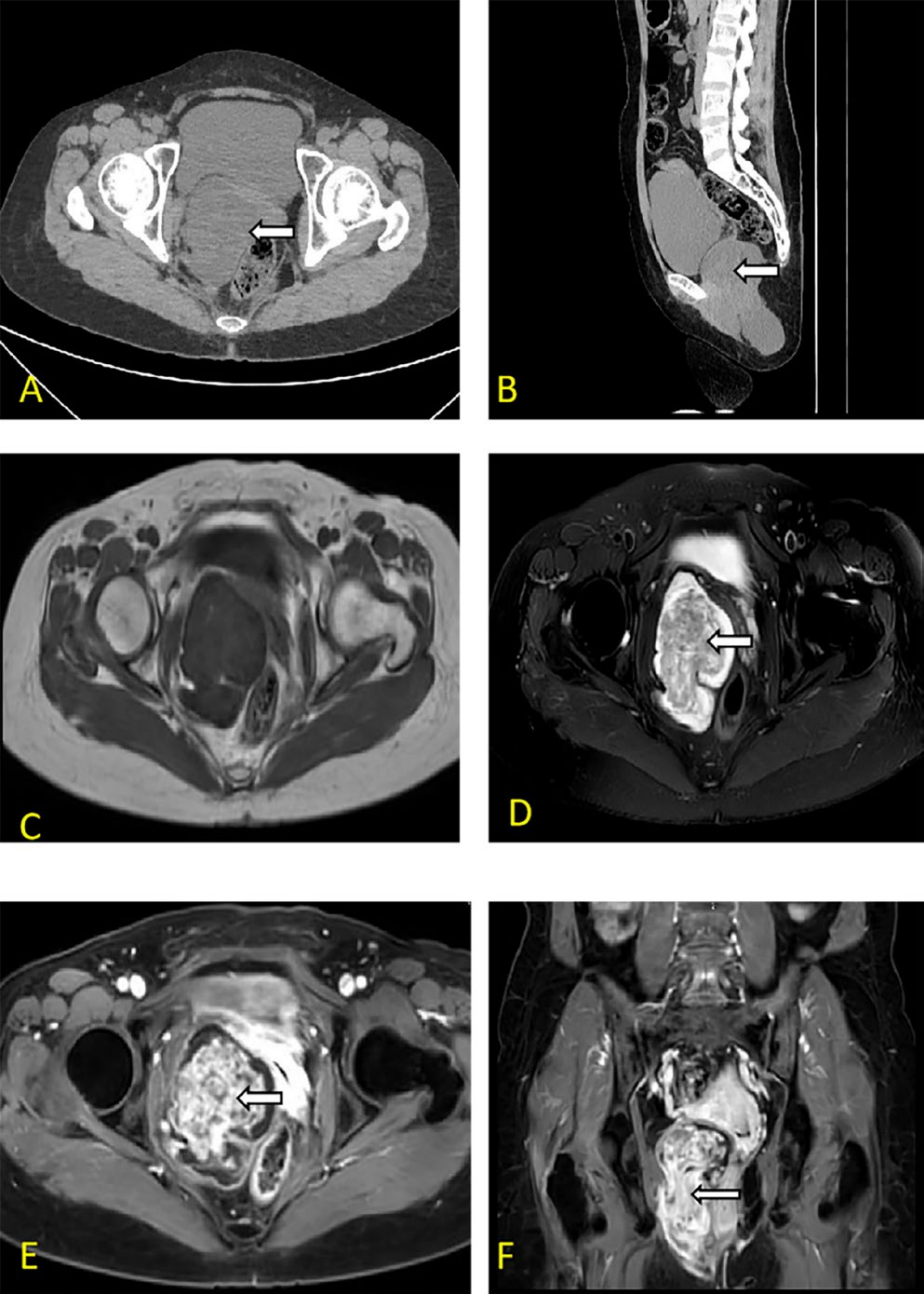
Patient's CT and MRI image. A large irregular mass on the right side of the pelvic floor extended through pelvic diaphragm into the ischiorectal fossa, displacing the rectum, uterus and vagina without invading. (A) Unenhanced CT image showed large pelvic mass being hypo‐attenuated in relation to surrounding muscular tissue. (B) Sagittal reconstruction image of CT scan showed a pedunculated soft tissue mass extending from the pelvic down to the subcutaneous soft tissue of the right buttock. (C) Axial T1‐weighted image showed a large pelvic floor mass was isointense and had a clear border to other tissue. (D) Axial fat‐saturated T2‐weighted imaging demonstrated a high‐signal intensity mass with inhomogeneity can be seen, and within it, there are streaky low‐signal intensity shadows running in bundles, showing its “layered” or “whirlpool” arrangement characteristics (white arrow). (E) Axial T1‐weighted post‐contrast image showed on the T2WI fat‐suppressed sequence, a high‐signal intensity mass with inhomogeneity can be seen, and within it, there are streaky low‐signal intensity shadows running in bundles, showing its “layered” or “whirlpool” arrangement characteristics. (F) Coronal T1‐weighted post‐contrast image revealed its “layered” or “whirlpool” arrangement characteristics (white arrow).
